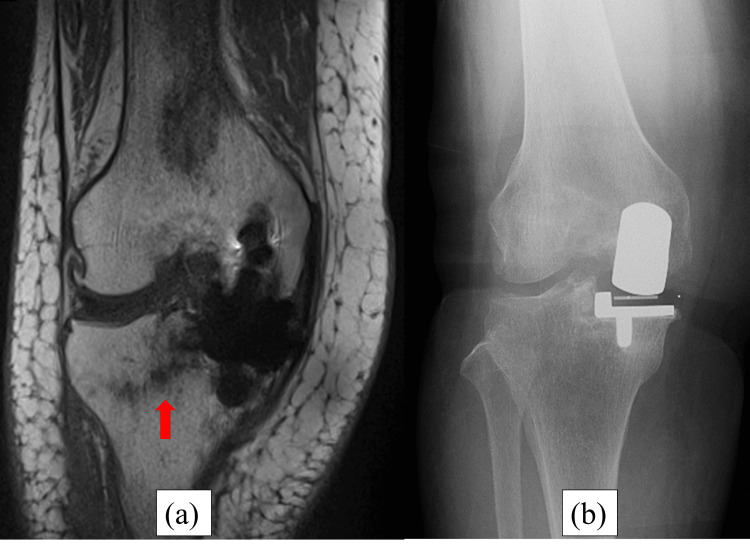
MRI and radiography at five weeks postoperatively. (a) Postoperative radiography shows no fracture line. (b) Postoperative CT shows the level of a pinhole. The red arrows show the place of the pin. The pin was placed on the tibial mechanical axis.
